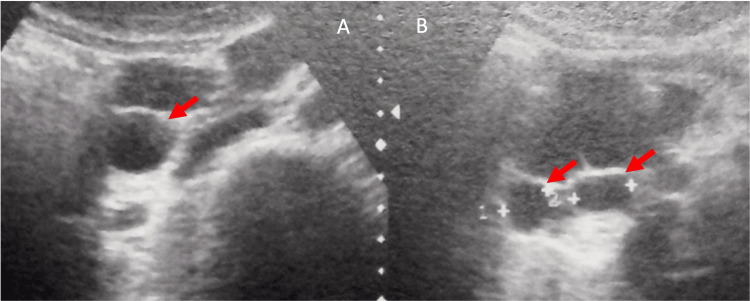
(A and B) Abdominal ultrasound: Several anechoic images, with rear acoustic reinforcement located in the head of the pancreas (red arrows).
